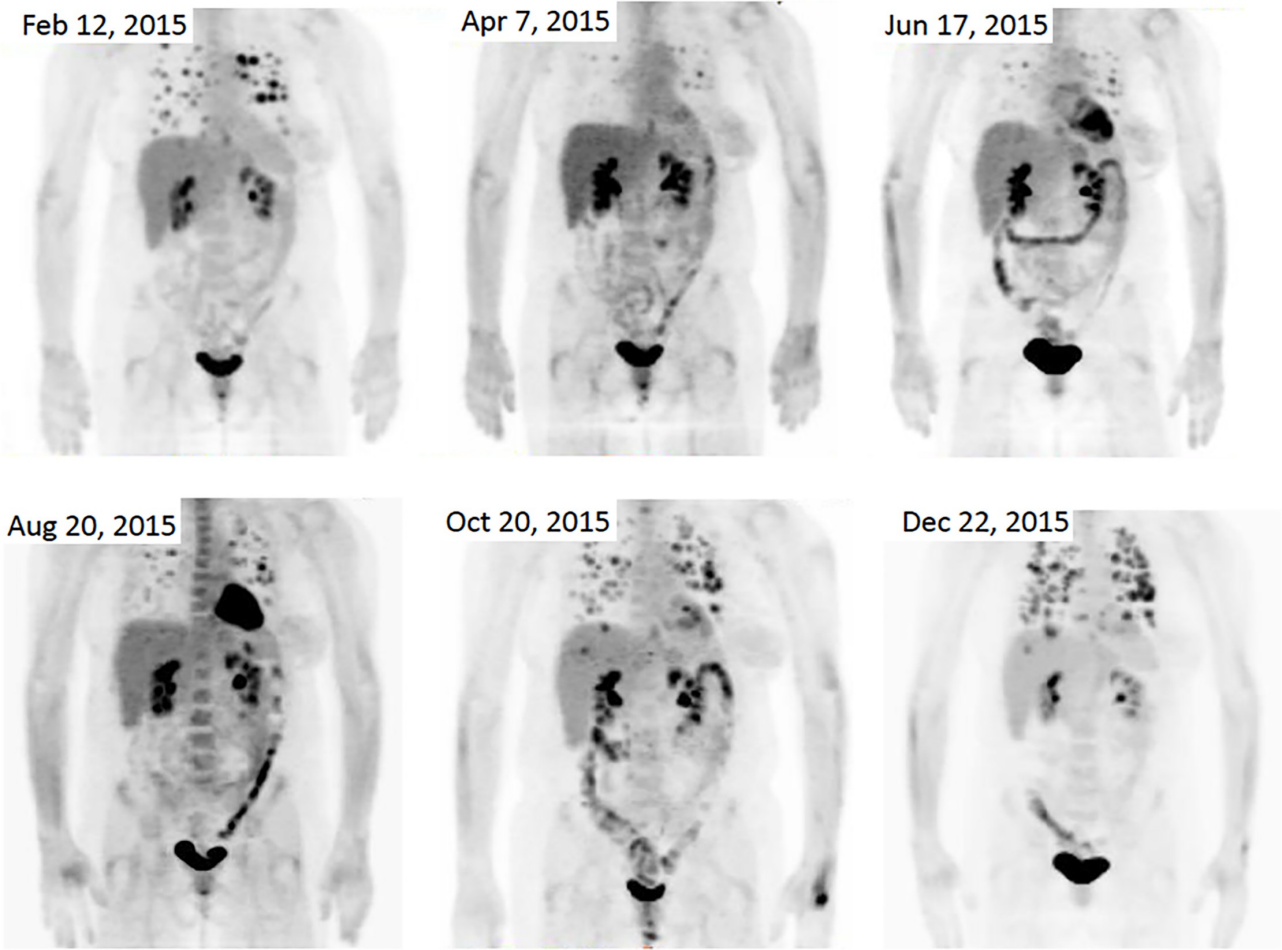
Treatment response by PET-CT. (A) Baseline imaging demonstrating pulmonary metastases (February 2015); (B) Imaging after 2 months of treatment with everolimus, demonstrating tumor regression (April 2015); (C, D) Persistent responses; (E, F) Disease progression.
